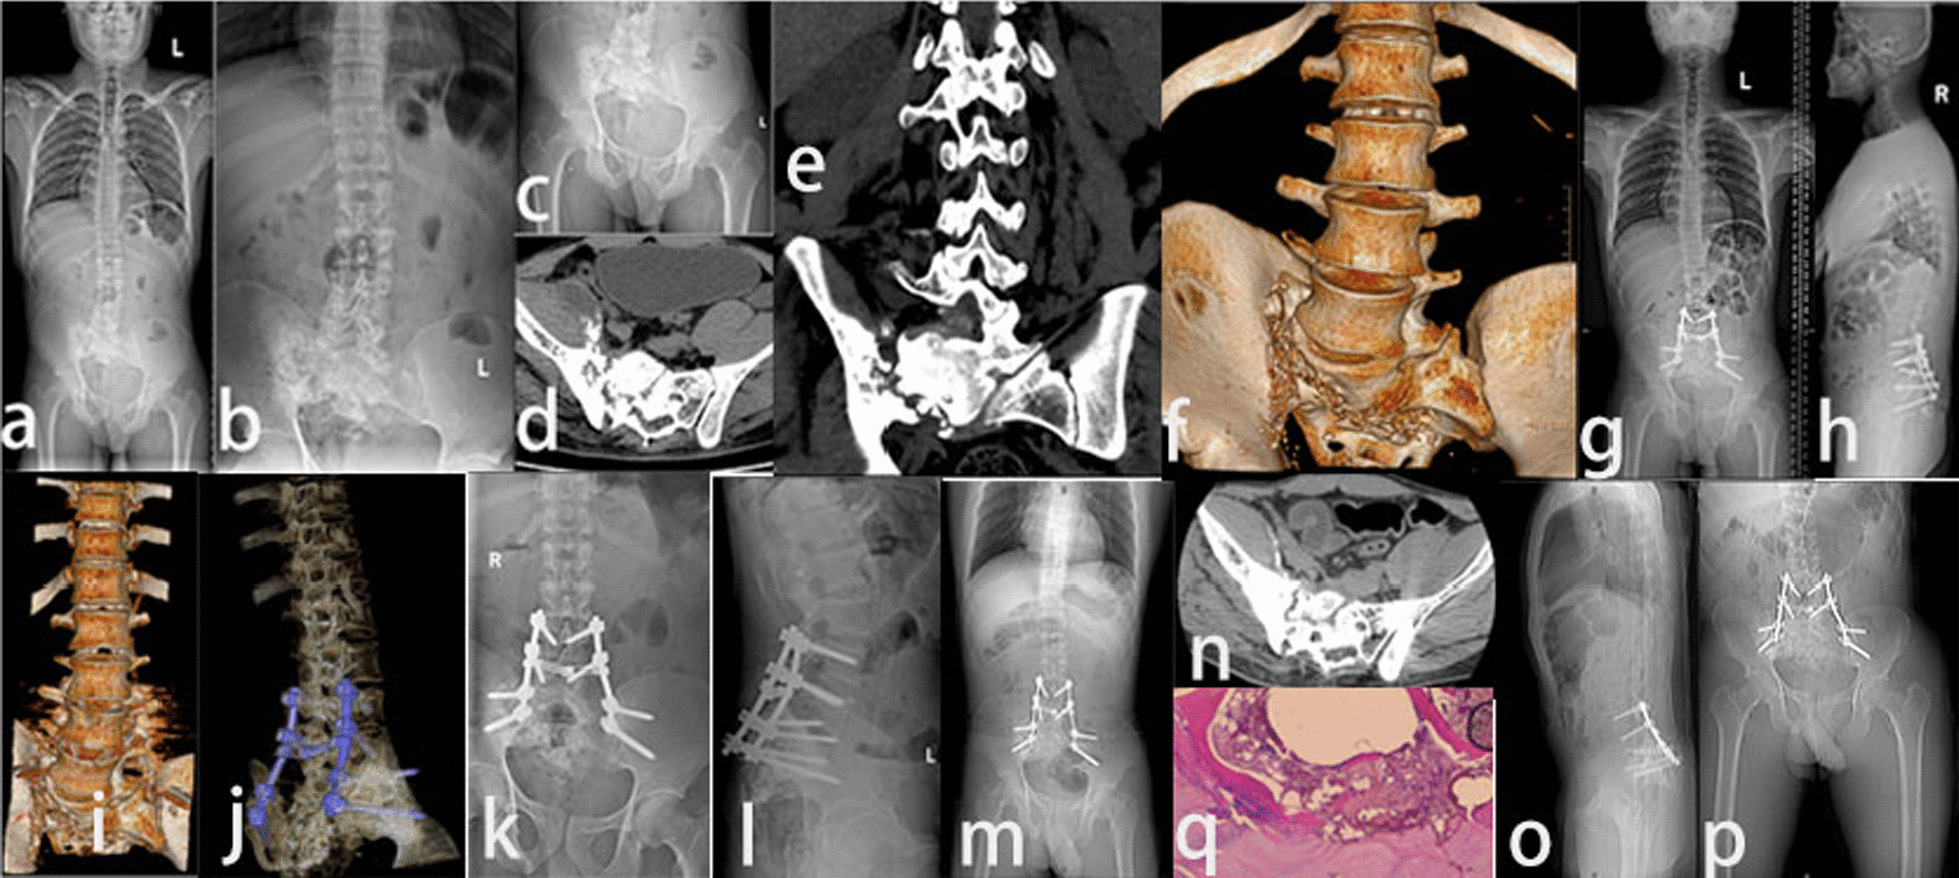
X-rays and CT before and after surgery of NO.5. a–c Preoperative X-rays d–f Preoperative CT showed the joint space disappeared. Strip calcification can be seen in the spinal canal behind the lumbar 4–5 vertebral body, and the spinal canal is narrowed behind the corresponding intervertebral space. The bone density of the right sacroiliac joint and sacrum is uneven, and multiple worm-like bone destructions can be seen, and multiple spot-like high-density shadows can be seen inside. Similar oval, slightly hypodense shadows in the right psoas muscle, multiple punctate calcifications can be seen on the edge of the lesion and in its interior. The right iliacus muscle was swollen, and punctate high density was seen in the musculature around the pelvic floor. g, h Postoperative X-ray. i, j postoperative CT. k, l X-rays 6 months after surgery. m, n CT 1 year after operation: the sacroiliac joint is fused, and the joint space disappears. o, p CT and X-ray films 2 years after surgery showed irregular shape of the right sacroiliac joint, fusion of the sacroiliac joint and disappearance of the joint space. q Examination results of surgically removed pathological tissue
